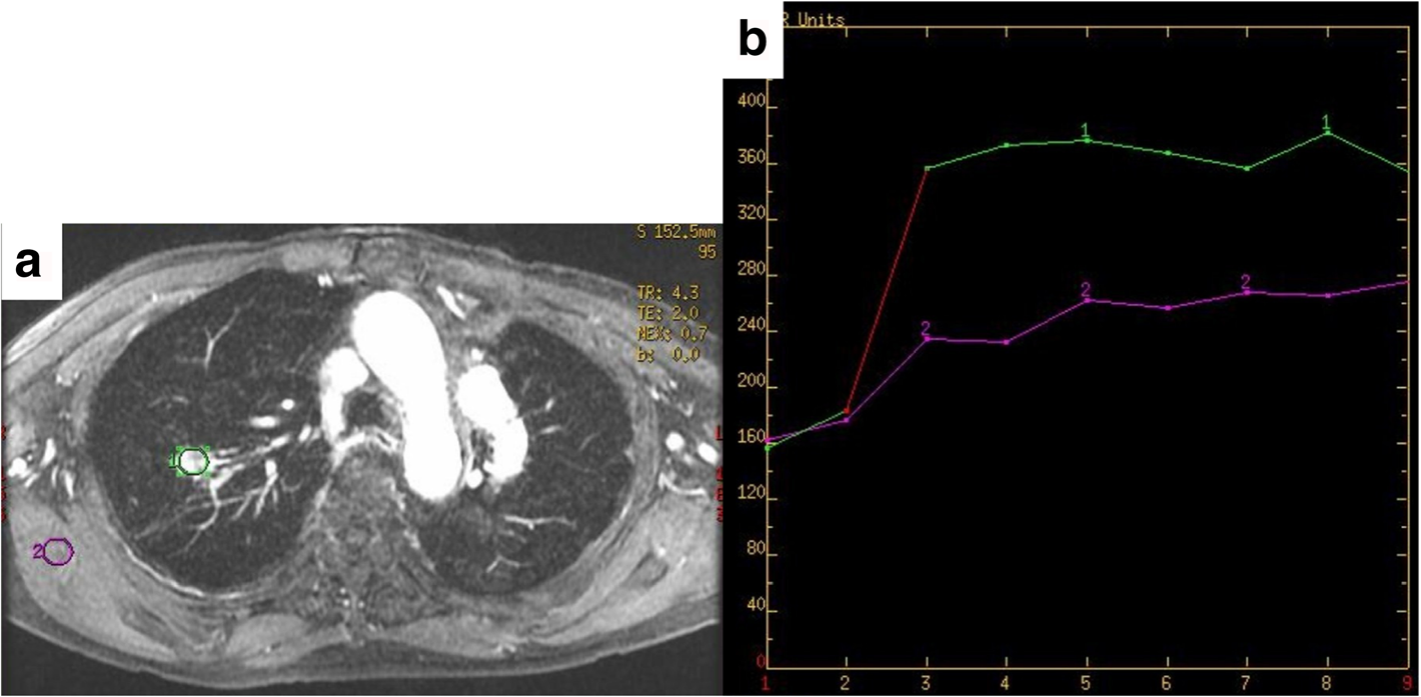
a Dynamic magnetic resonance imaging showing contrast enhancement effect in the solid nodule on the cut-end of the residual right upper lobe. b Graph showing time-intensity curve compared to normal muscular tissue of the chest wall
